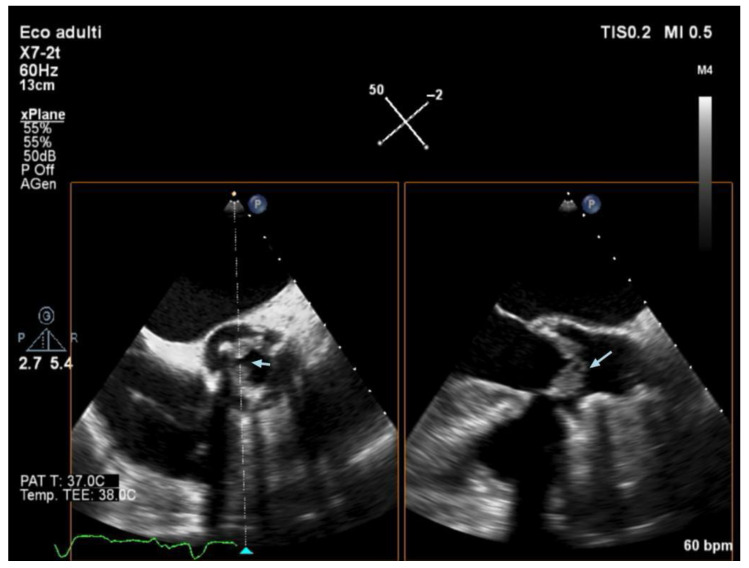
Transesophageal echocardiography—midesophageal aortic valve short axis (left) and long view (right) obtained with X-plane. Aortic valve bioprosthesis with evidence of irregular isohyporeflective thickening of the leaflets due to the presence of mobile vegetative lesions of an endocarditic nature.
